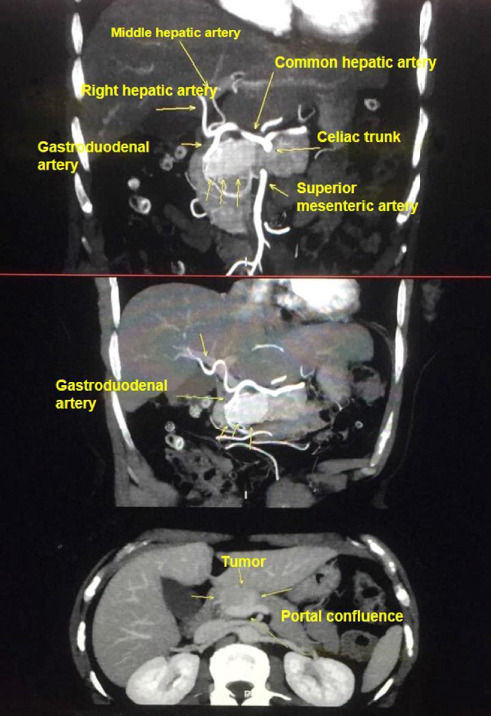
Contrast-enhanced magnetic resonance imaging, showing a 3.5 × 2.2 × 1.9 cm hypervascular solid mass at the head and body of the pancreas.
